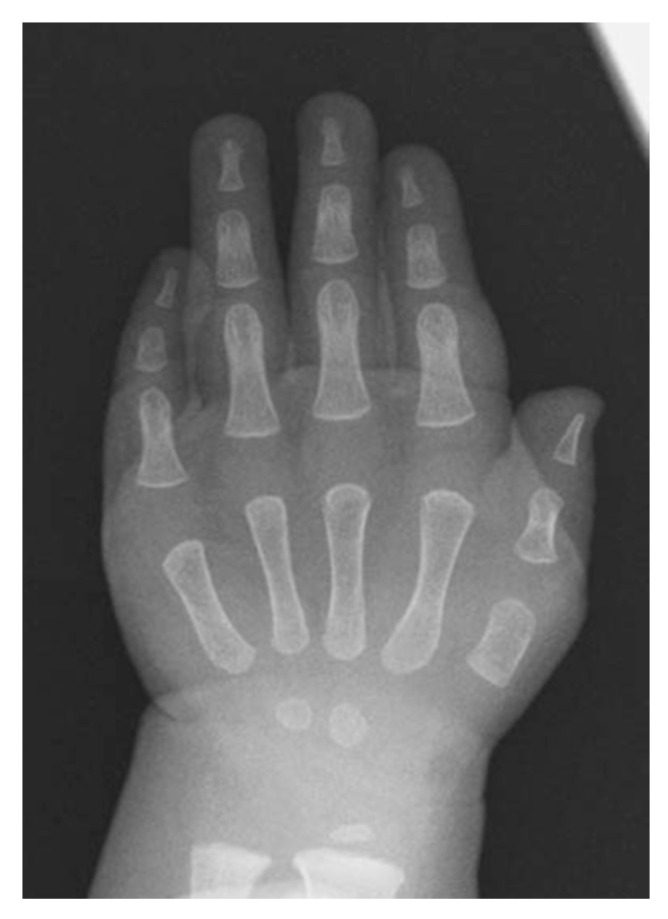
Anteroposterior radiograph of the left hand of a FOP boy at the age of 11 months demonstrating marked shortening of the first metacarpal bone.
